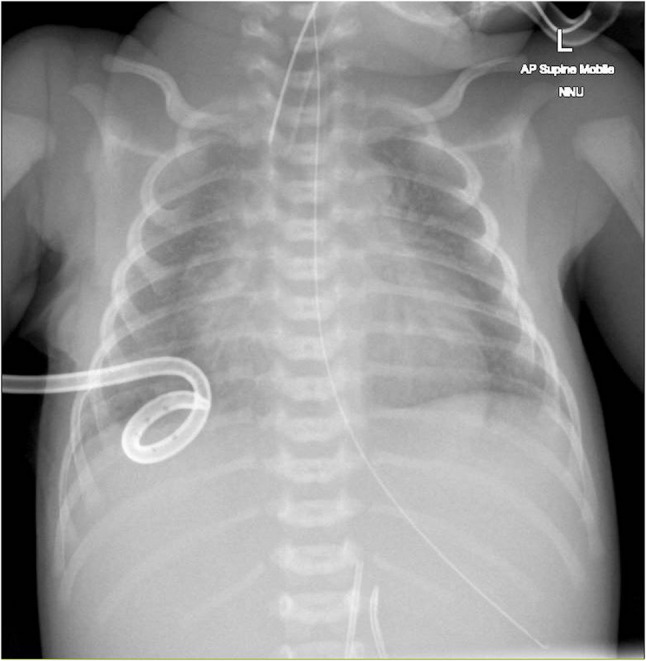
Admission Chest X-ray following chest drain insertion. UVC and UAC were re-inserted due to suboptimal placement
